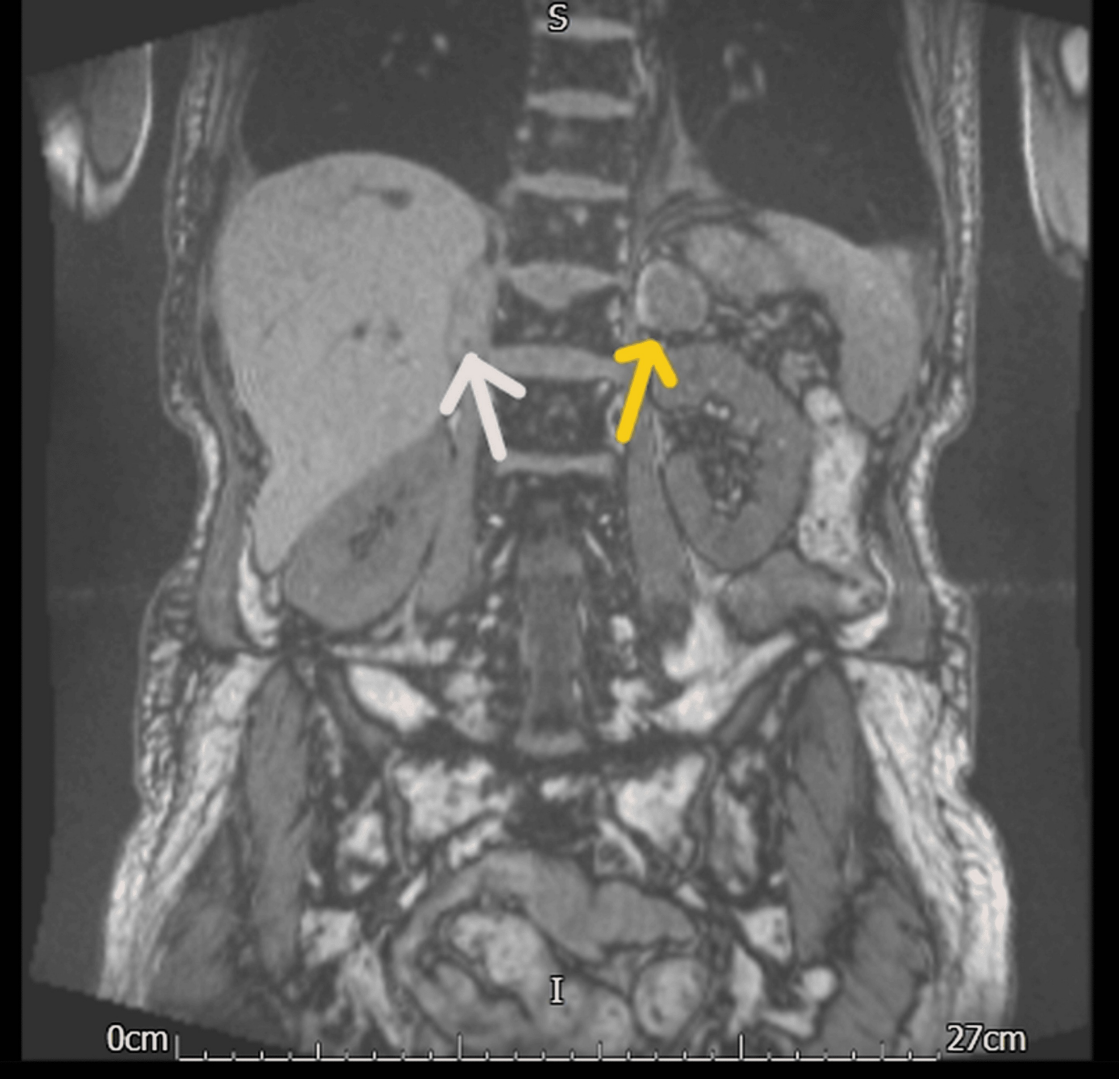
MRI of the adrenal glands MRI adrenal gland (coronal T1 weighted) is showing both adrenal glands are enlarged, the left one (the yellow arrow indicating the left adrenal gland) being larger. Both glands are ellipsoid/rounded in shape rather than the typical bifid appearance. The left adrenal gland measures a maximum size of 38 mm; the right adrenal gland (the pink arrow is showing the right adrenal gland) measures approximately 27 mm in maximum diameter.
